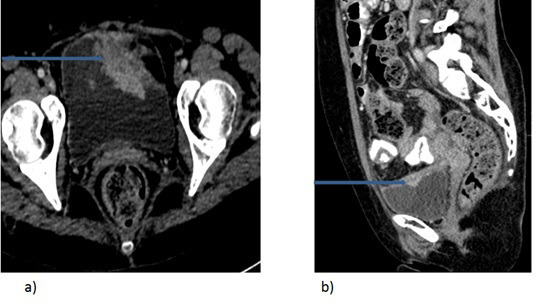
A 54-year-old female with breast cancer presenting with hematuria. Axial (a) and sagittal (b) CT images of the of the pelvis show enhancing irregular bladder wall thickening (arrows).
